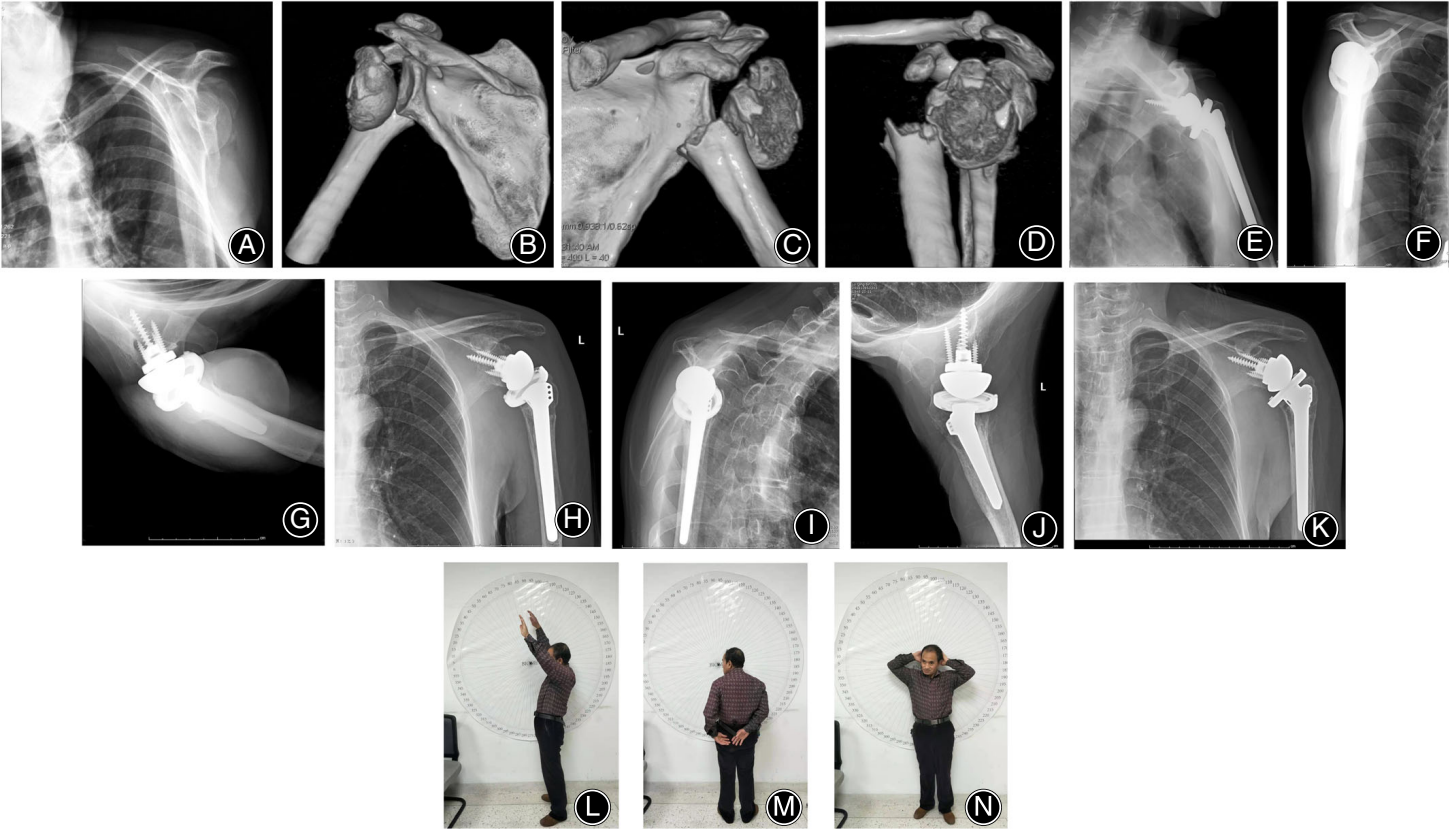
A 70 year‐old male patient with left proximal humeral comminuted fracture (Neer three part fracture) caused by a fall. (A–D). X‐rays and 3D‐CT showed comminuted fracture of proximal humerus with serious compress fracture of the greater tuberosity. (E–G). The X‐rays in the AP view, Y view and axillary view indicated that the prosthesis position was satisfactory after the reverse shoulder arthroplasty. (H–J). At the 5 months follow‐up after operation, the X‐ray films of the AP view, Y view and axillary view showed satisfactory healing of the greater and lesser tuberosities. (K). At the 7 months follow‐up after operation, the AP view indicated that the prosthesis position was satisfactory. (L‐N). At the 7 months follow‐up after operation, the forward elevation was 150°; external rotation was 30°; internal rotation was 40°. VAS score was 0°; The Neer score was 94; Constant‐Murley score was 96.
